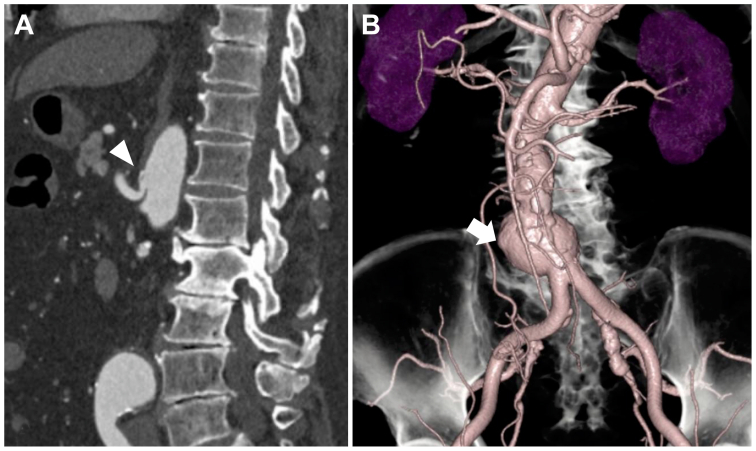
(A) Sagittal computed tomography (CT) scan revealing celiac artery (CA) stenosis (*white arrowhead*) caused by the median arcuate ligament. (B) Three-dimensional CT scan demonstrating an infrarenal abdominal aortic aneurysm (AAA) with a maximal diameter of 51 mm (*white arrow*).
